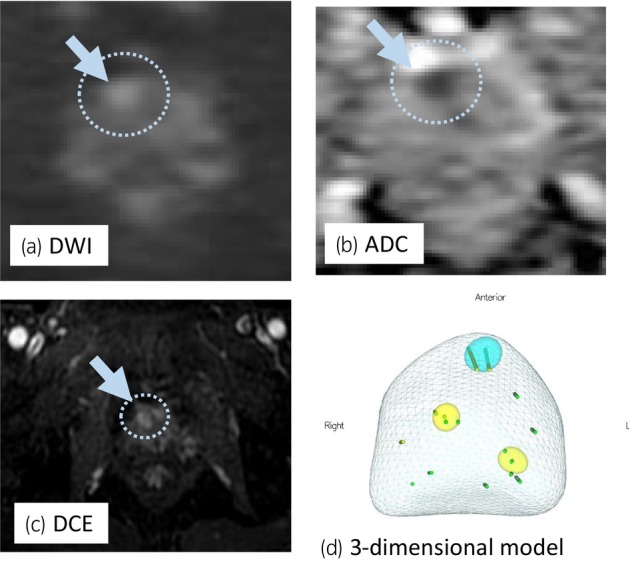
Targeted lesion of FT in pre‐operative MRI. Multiparametric MRI revealed PIRADS‐category 4 lesion in right anterior TZ with 13 mm in diameter (a–c; blue arrow and circle). MR/US fusion targeted biopsy found GG 1 (blue lesion, in d). No cancer were seen in other targeted biopsies (yellow lesions, in d) and any systematic biopsies.
